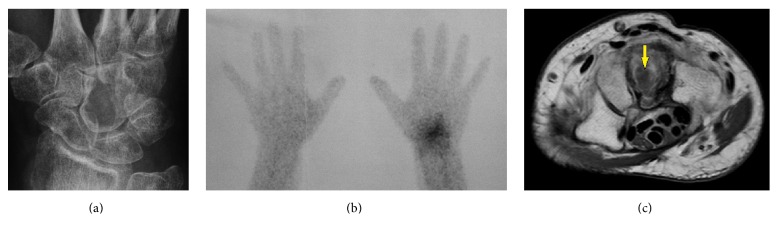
Case report (preoperative diagnostic findings): (a) PA radiograph showing lytic lesion in capitate bone with cortical thinning and cortical destruction distally; (b) bone-granulocyte scintigraphy of both hands showing increased radionuclide uptake in the region of right carpal bones 45 minutes after application; (c) axial MRI sequence showing low to intermediate signal intensity in capitate bone and no soft-tissue extension* (arrow)*.
